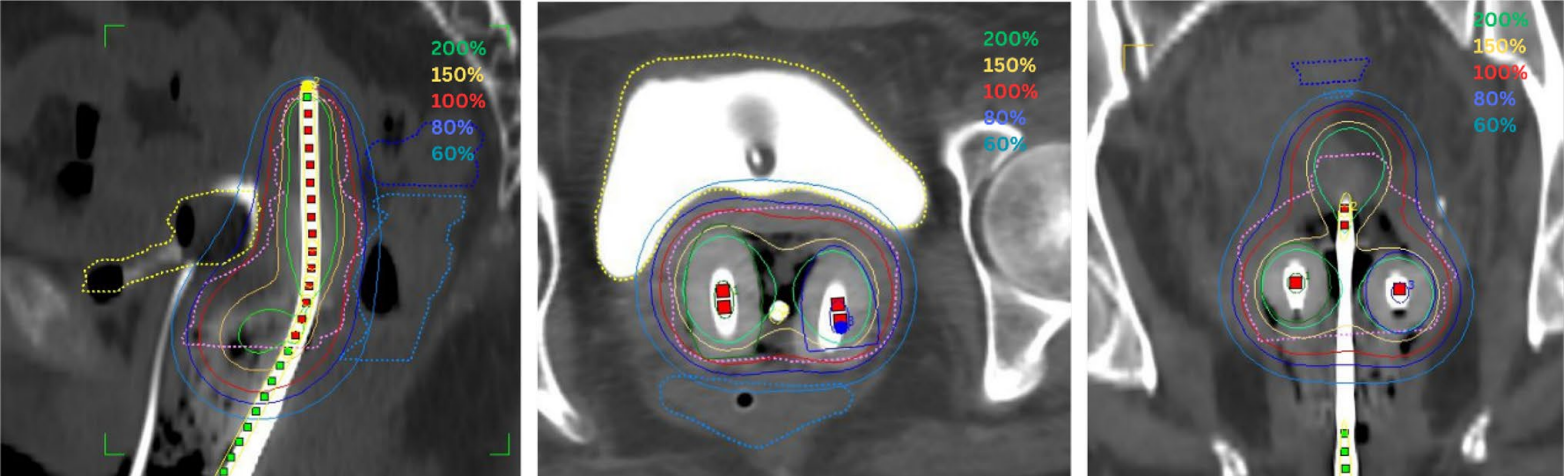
CT scan with Brachytherapy applicator in situ
